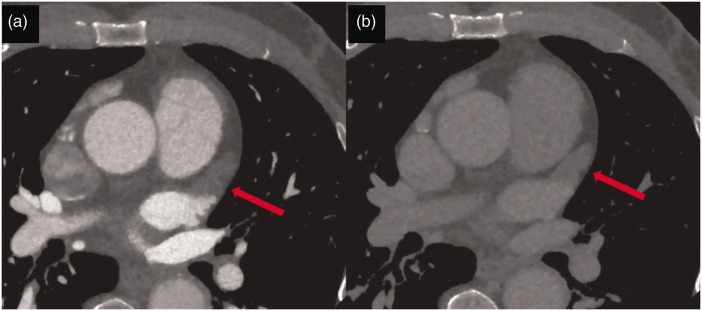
(a) Axial cardiac computed tomography image shows the presence of thrombus in the left atrial appendage (red arrow) and (b) A late scan showing persistence of low attenuation at a repeat scan 60 s after the first scan (used with permission).
